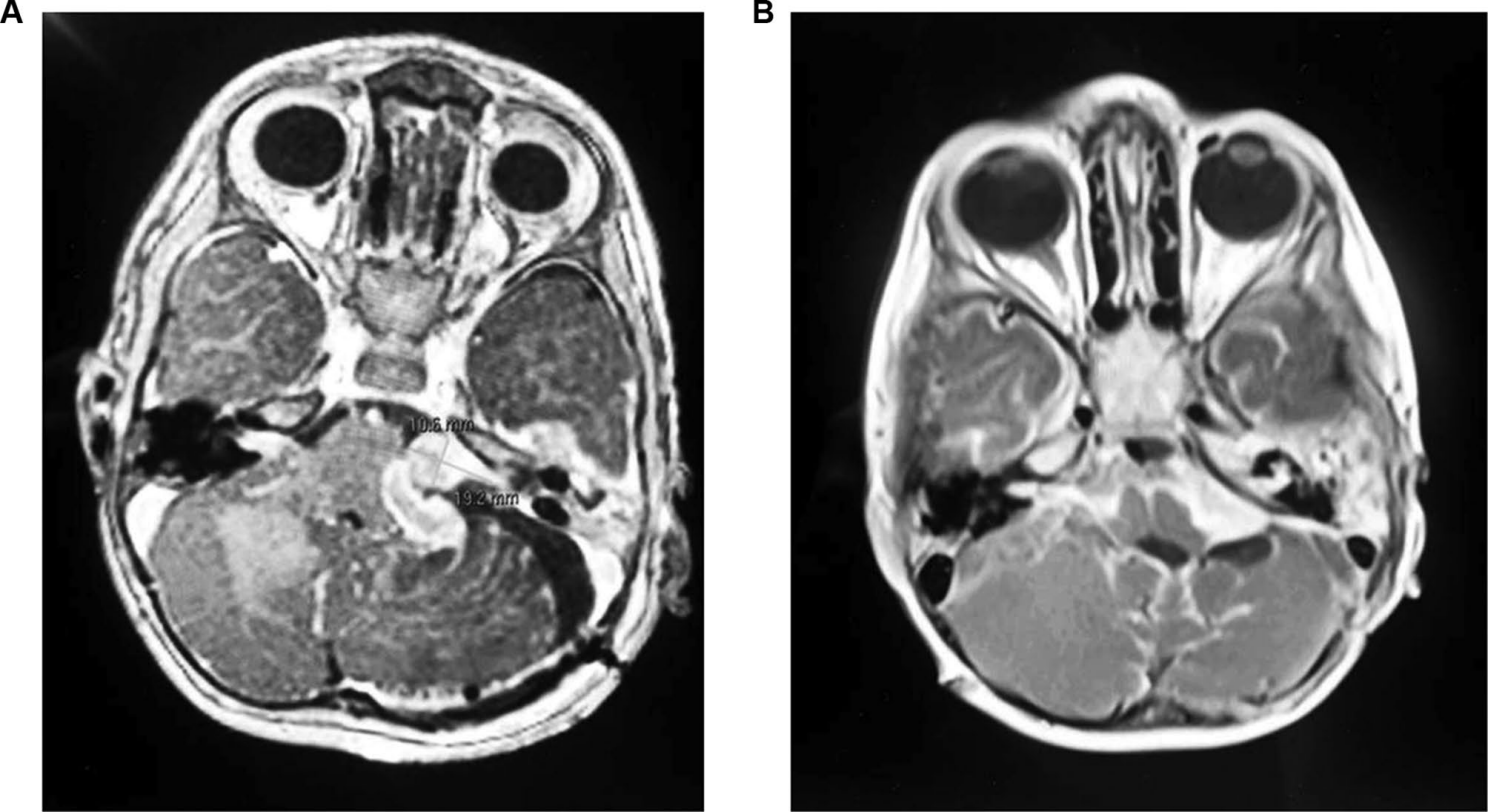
a Post operative MRI Brain with tumour remnant on the left side. b MRI demonstrating lesion progression after chemotherapy bilaterally
